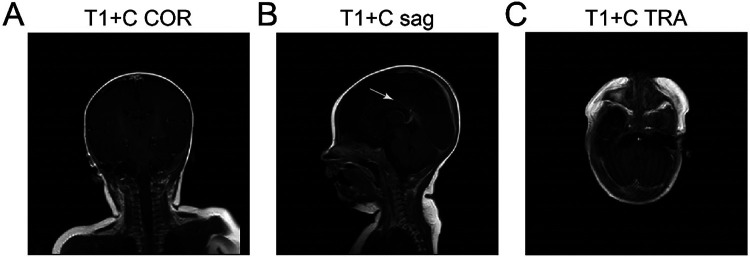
Contrast-enhanced brain MRI on April 6, 2025. (A) Coronal T1-weighted postcontrast image. (B) Sagittal T1-weighted postcontrast image. (C) Axial T1-weighted postcontrast image.
